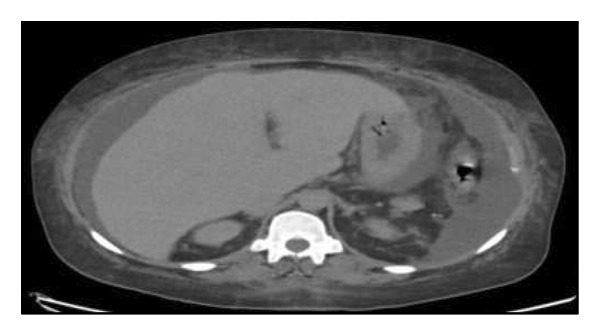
Abdomen and pelvis CT scan, revealing moderate ascites and irregular liver contours.
